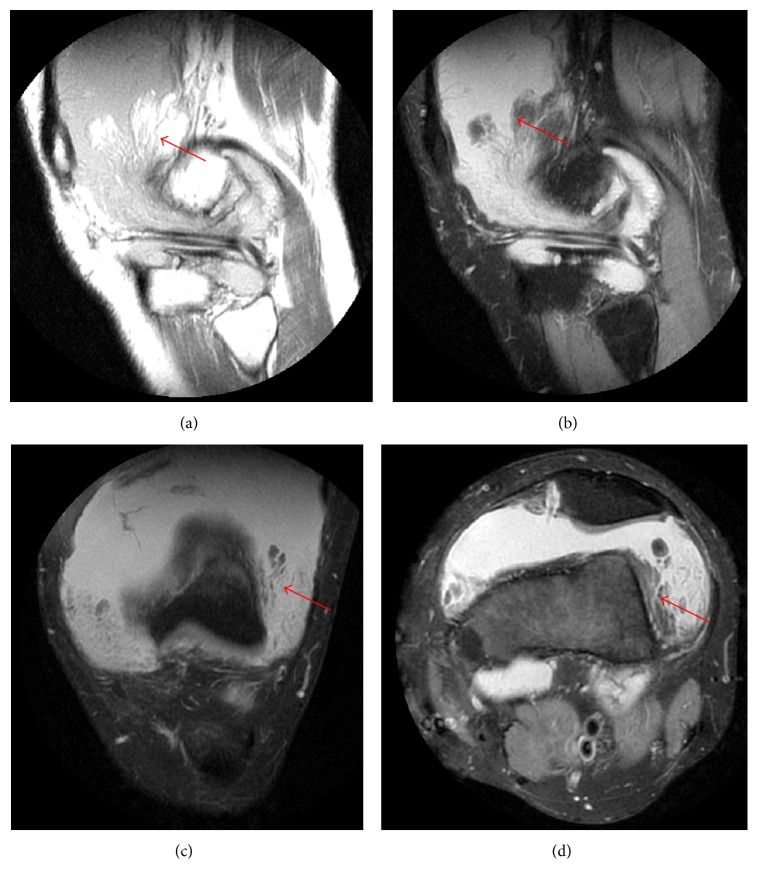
MRI of a 22-year-old male patient with lipoma arborescens of knee joints. Sagittal noncontrast T1 (b), weighted image, showed high signal intensity frond like synovial thickening in the suprapatellar bursal region (arrow) with joint effusion. GE HDesigna 3.0 Tesla magnetic resonance imaging system TR-737ms TE-9.3ms 3.5 mm slice thickness with interslice gap of 1 mm was used. Sagittal (a), coronal (c), and axial (d) fat suppressed proton density images revealed complete suppression of signal intensity of villous projections (arrow) in suprapatellar bursal region. GE HDesigna 3.0 Tesla magnetic resonance imaging system was used. Sagittal TR-2264ms TE-24ms 3.5 mm slice thickness with interslice gap of 1 mm, coronal TR-2740 TE-24 3.5 mm slice thickness with interslice gap of 0.5 mm, and axial TR-1956 TE-22.8 4 mm slice thickness with interslice gap of 1 mm.
